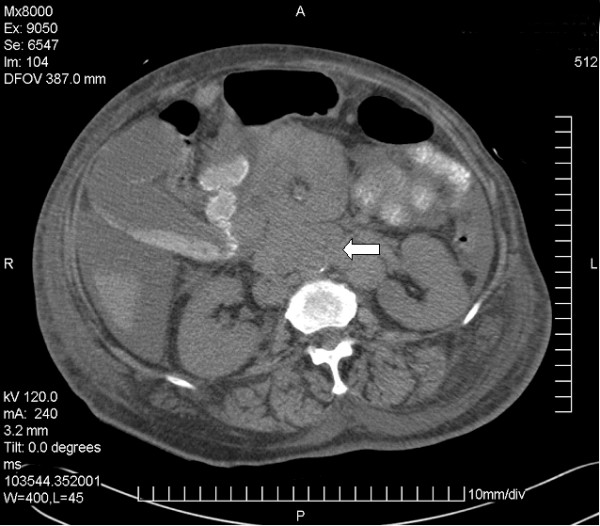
CT of upper abdomen. Extensive lymphadenopathy is noted in the para-aortic area (arrow) and surrounding the superior mesenteric artery. Body wall oedema, ascites and gall bladder sludge reflect the patient's debilitated condition.
